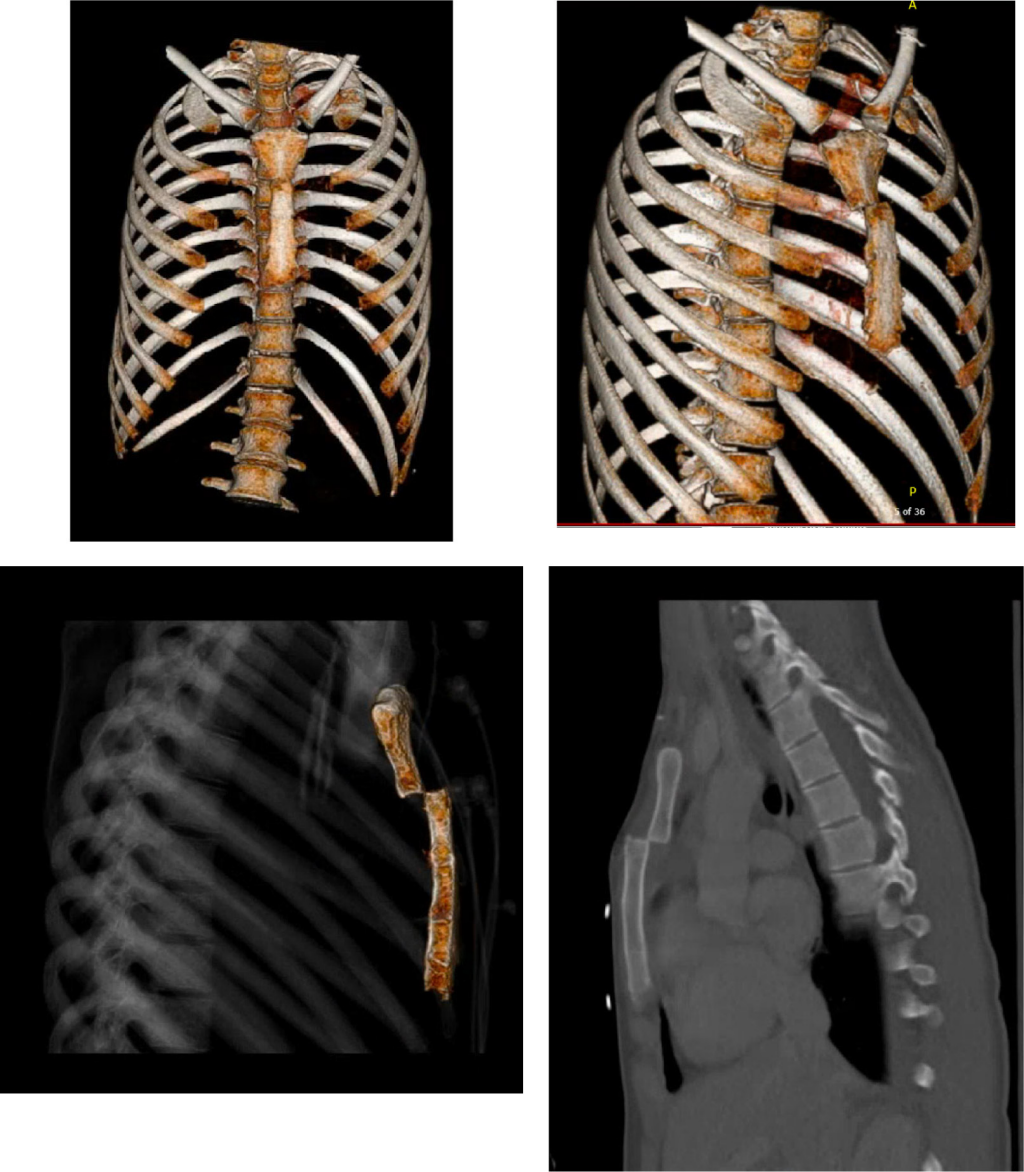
CT 3‐D reconstruction of fully displaced sternomanubrial fracture from blunt chest trauma.
